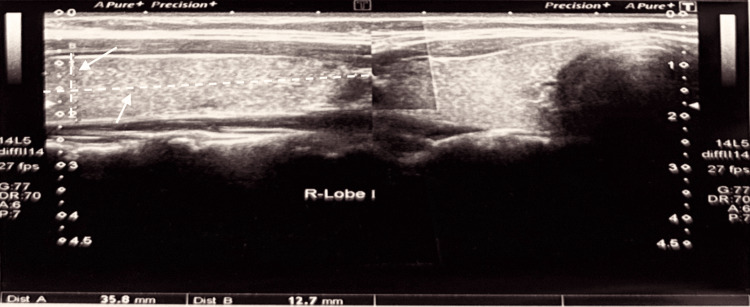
Thyroid ultrasound of a 22-year-old female showing the size of the right lobe of the thyroid, measuring 3.5 × 1.2 cm.
